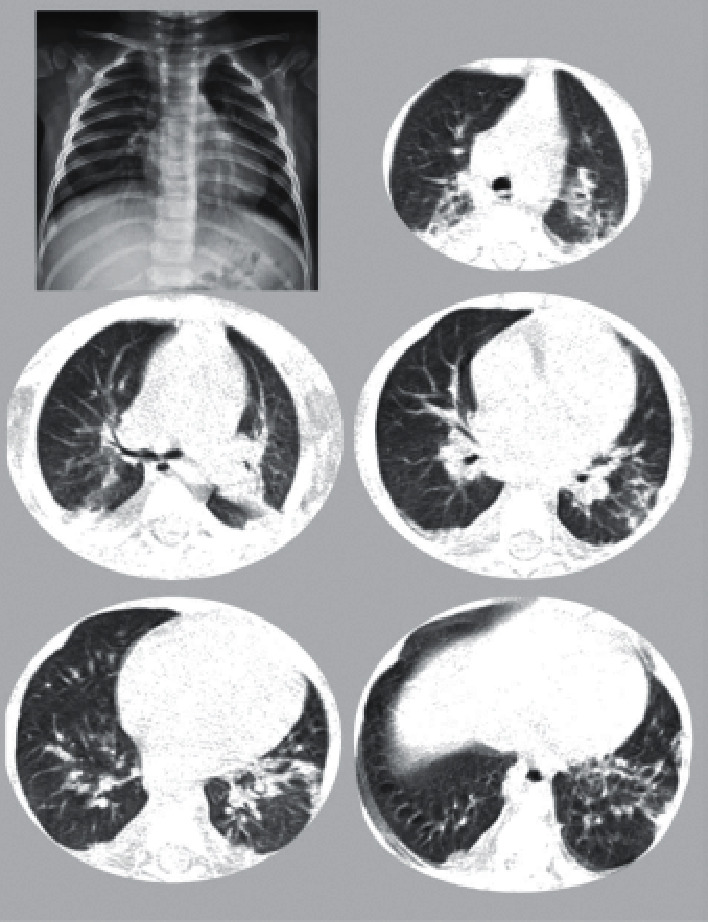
Chest X-ray and CT scan on the 3^rd^ day of admission show bilateral ground-glass opacities.
